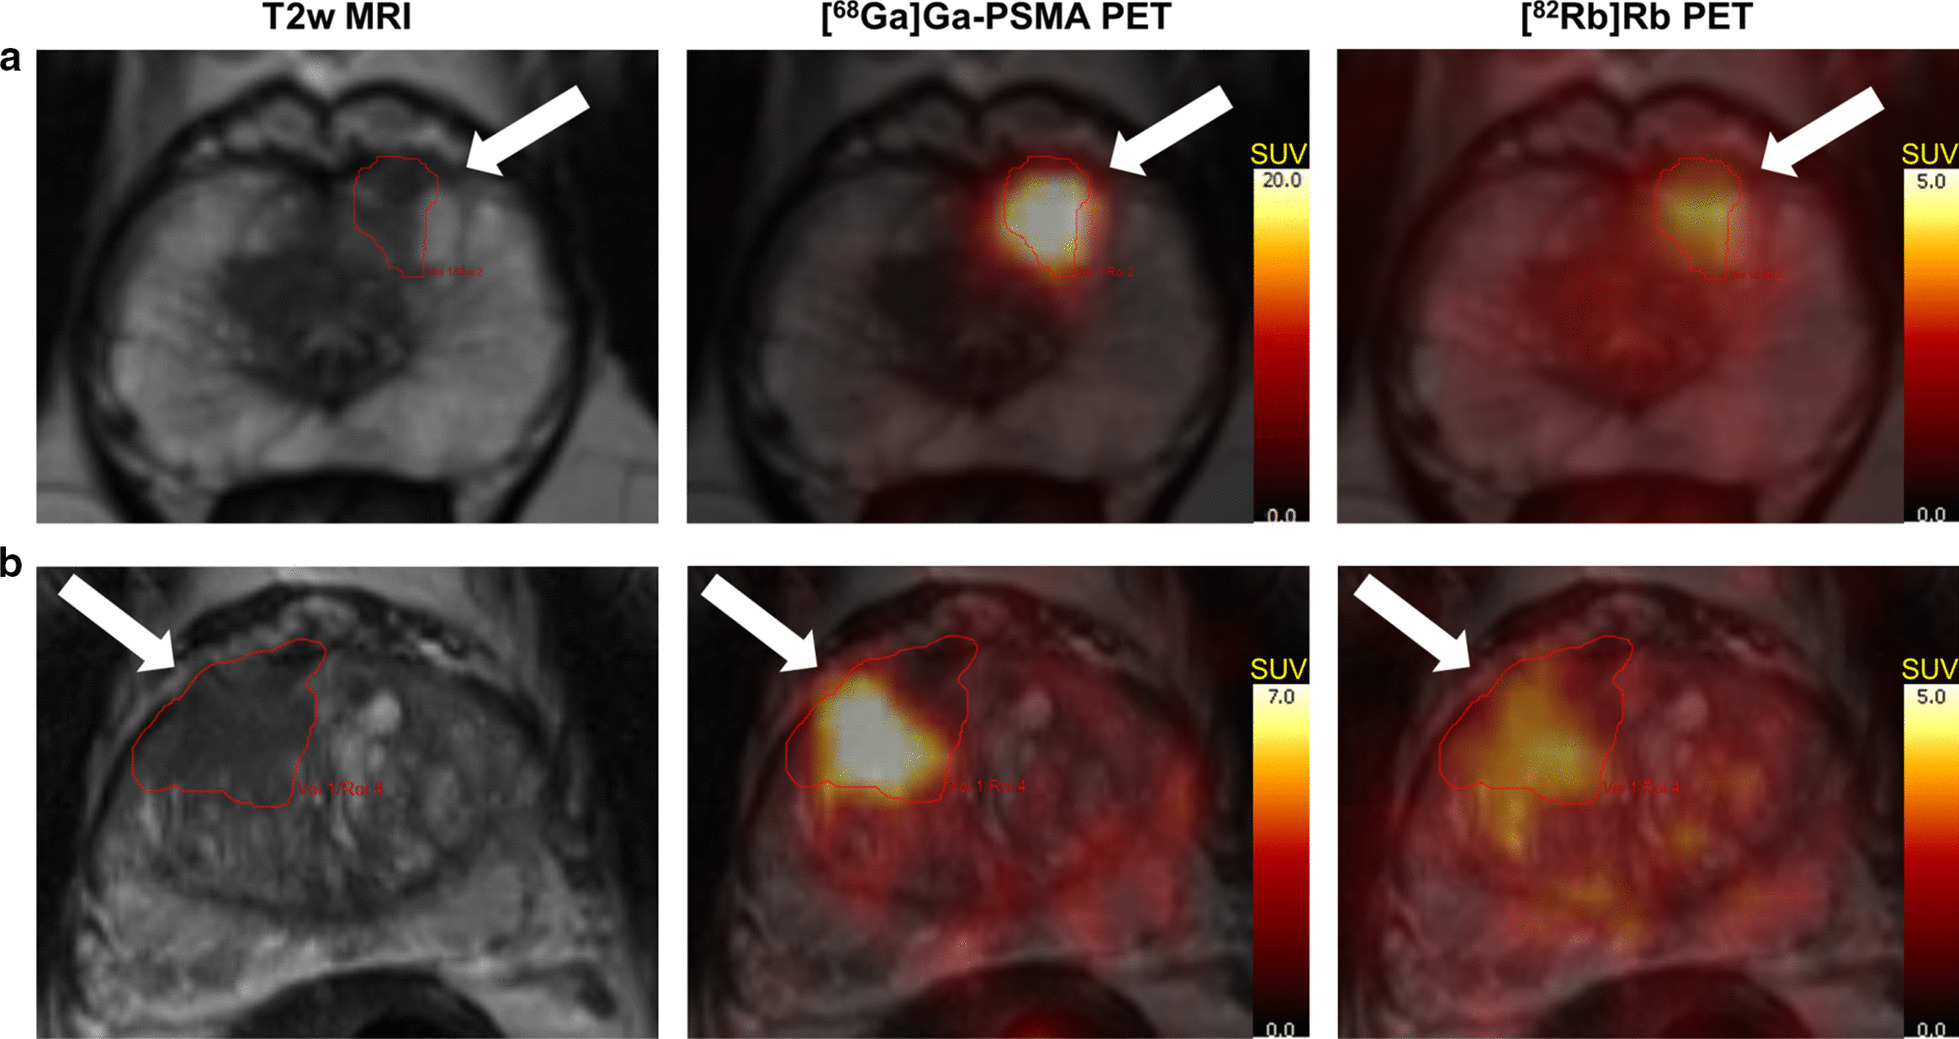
Demonstration of a MRI-guided tumour VOI with similar high uptake patterns of both [^68^Ga]Ga-PSMA-11 and [^82^Rb]Rb (a) and an example of inhomogeneous tracer distribution within the MRI-guided tumour VOI (b)
